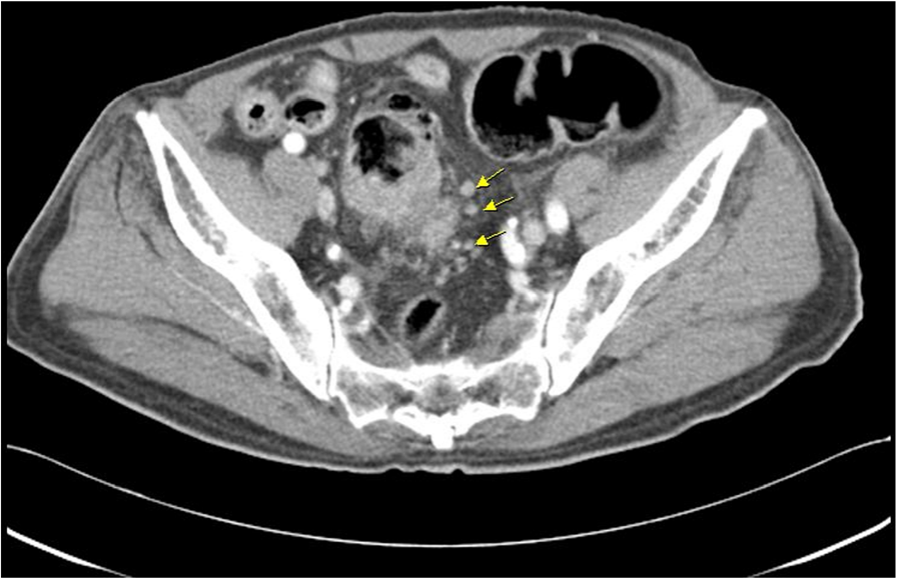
Computed tomography of abdomen shows huge sigmoid colon cancer. Arrows indicate enlarged peri colic lymph node
